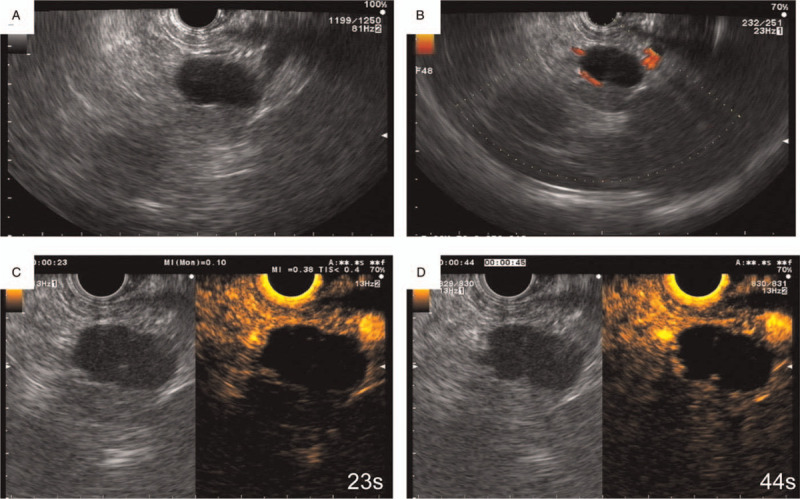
EUS images showing a pancreatic mass located in the tail. (A) B-mode and (B) color Doppler. Contrast-enhanced EUS demonstrating the different time (C: 23 s and D: 44 s) of blood perfusion. EUS = endoscopic ultrasound
